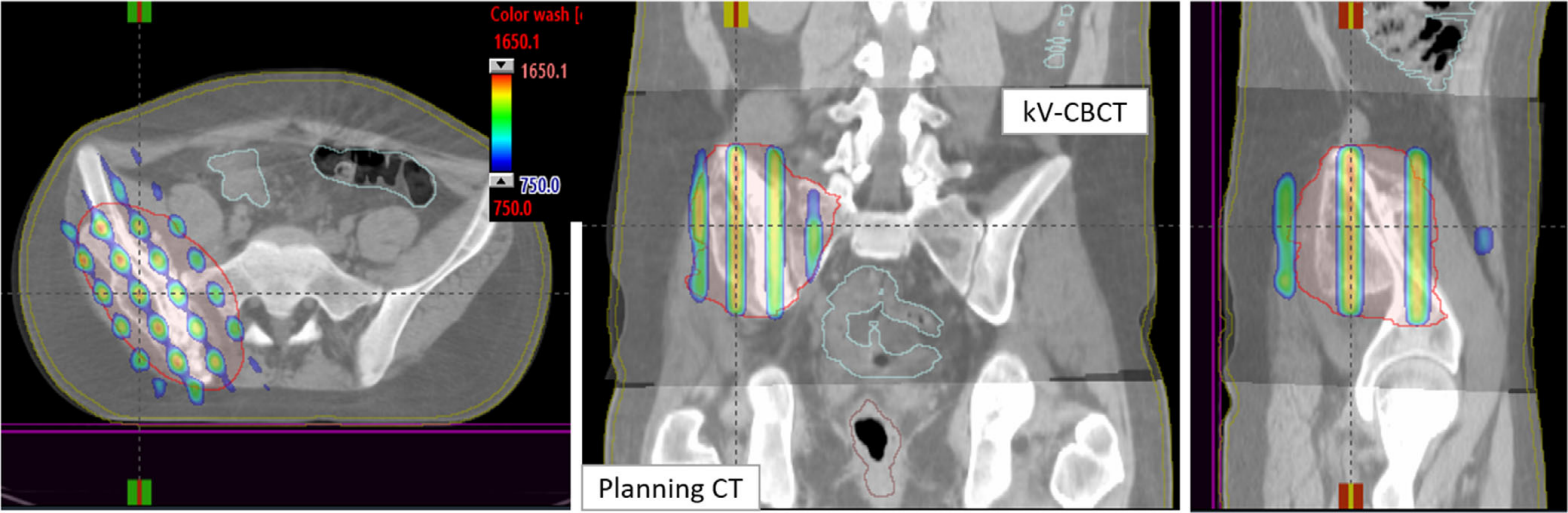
Demonstration of axial, coronal, and sagittal views of kV‐CBCT images (see the inset) coregistered with planning CT images (see the back of coronal and sagittal views) used for CBCT‐guided SFRT treatment on TrueBeam Linac. The overlaid planned isodose colorwash (50%–110%) with anatomical landmarks is shown for a patient treated with MLC‐based 3D‐conformal SFRT (15 Gy in one fraction) for a deep‐seated bulky mass of 10.0‐cm‐diameter tumor in a right pelvis—malignant neoplasma of connective and soft tissue of trunk. CBCT images were acquired in the treatment position followed by performing automatic rigid‐registration and manually fine‐tuning the registration for tumor soft‐tissue alignment before applying the couch correction. 3D, three‐dimensional; SFRT, spatially fractionated radiation therapy
